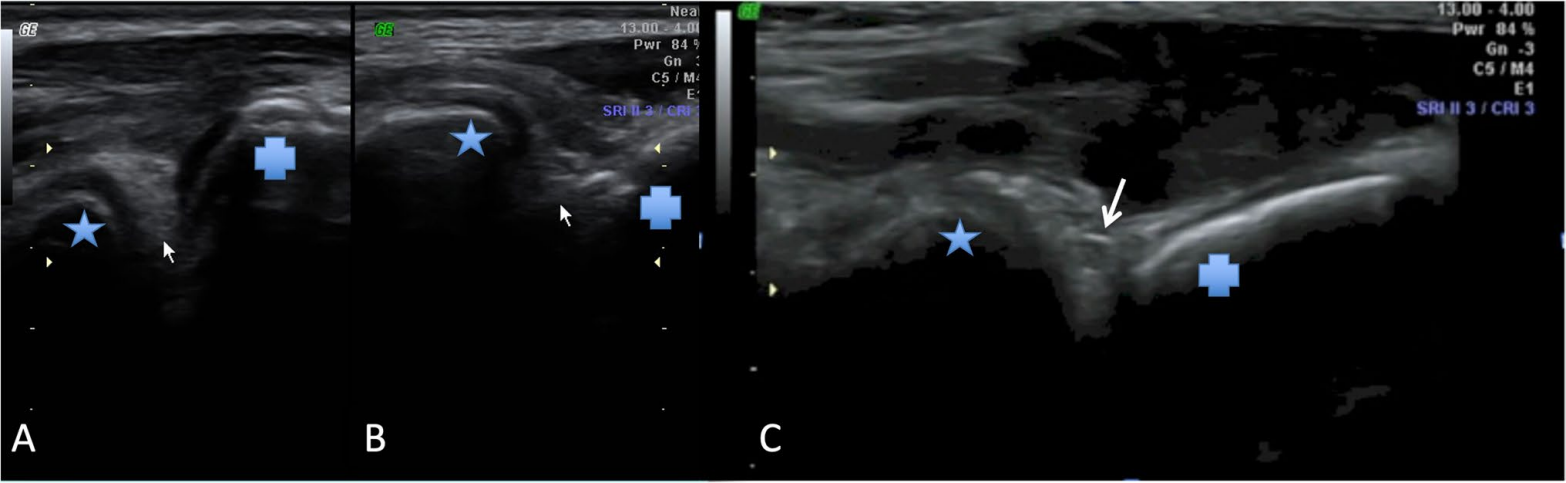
Presents ultrasonographic picture of lateral fold of the elbow. a and b Fibrotic fold (marked with short arrows) — a Fold in relaxed state and b compressed fold during flexion what was responsible for clinical symptoms. c Swollen fold with formation of accumulation of fluid — a cyst marked with an arrow. Cysts are typically formed in the peripheral areas of the fold where pressure excreted by bone margins are highest. Star presents lateral epicondyle of the humerus, cross marks lateral outline of radial head
